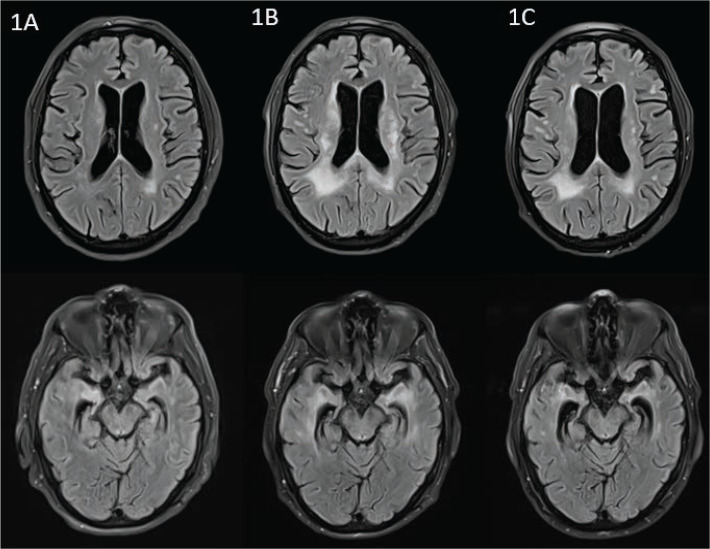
Α) MRI findings on presentation: T2-weighted and fluid-attenuated inversion recovery (FLAIR) images reveal hyperintensity in the medial temporal lobes, non-specific hyperintense small-diameter lesions in sub-cortical and deep white matter, Β) Cerebral MRI one month after initiation of corticosteroid therapy showing expansion of hyperintense areas in the medial temporal lobes with accompanying atrophy, increased lesion load in periventricular and deep white matter, C) Cerebral MRI two months later after initiating cyclophosphamide showing decreased lesion load in periventricular and deep white matter, attenuated hyperintensity in temporal lobes accompanied by significant atrophy.
